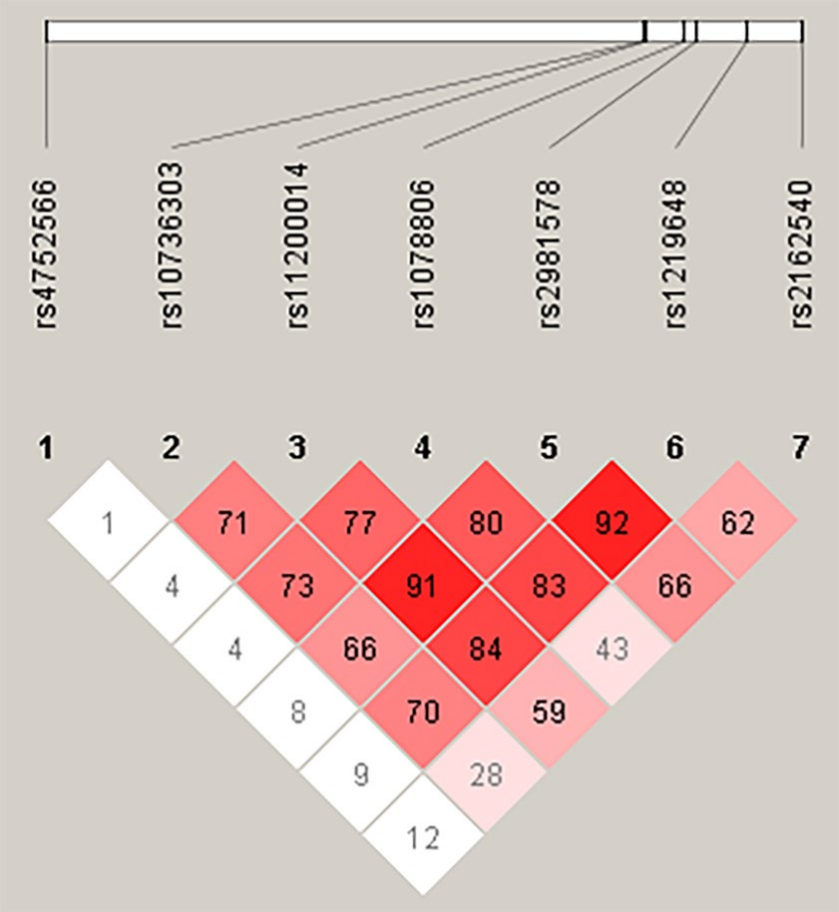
Box‐plot comparing SNB angle values among rs2981578 genotypes (CC, CT and TT). The box represents the interquartile range (IQR) and whiskers indicate 1.5 × IQR. The median SNB angle was lower in carriers of the T allele.
